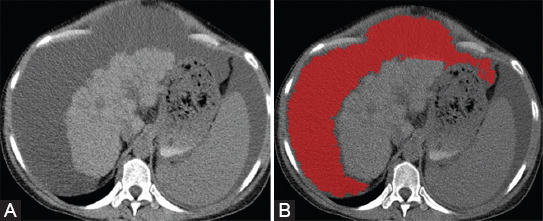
(A) Axial CT non-enhanced phase image of a 58-year-old patient with cirrhosis. (B) The slice with the region of interest (red area) used for texture analysis.
